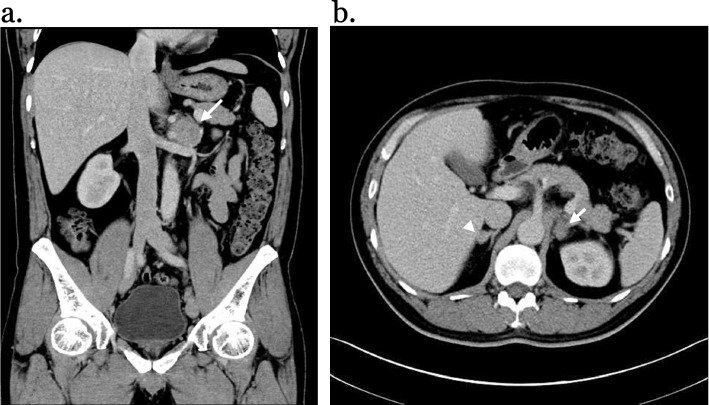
Abdominal computed tomography showed (a) one 4.3 cm left para-aortic tumor (arrow) surrounded by the aorta, celiac trunk, superior mesenteric artery, and renal vessels, with the Hounsfield unit being approximately 40 under the noncontrast phase and 70 under the arterial phase and (b) a 2.1 cm left adrenal tumor (arrow) and a 1.2 cm right adrenal tumor (arrowhead)
